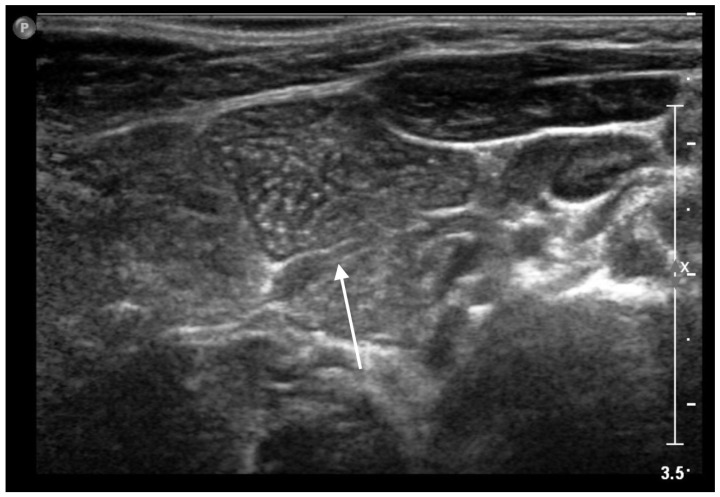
Follow-up ultrasound showing a slight reduction in size (2.6 × 1.7 cm) of the ectopic cervical thymus (*white arrow*).
